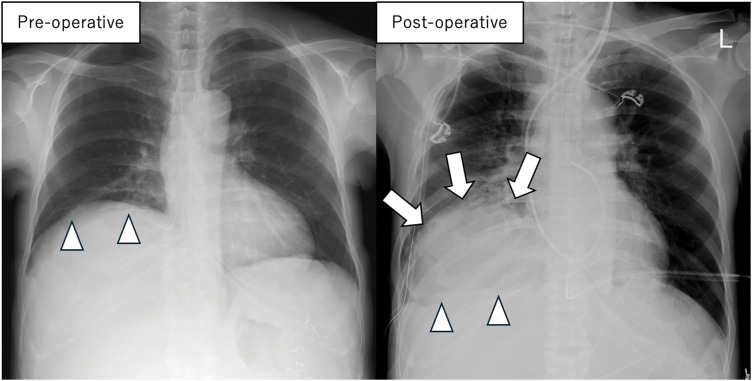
Comparison of preoperative and postoperative chest radiographs. The arrows indicate the pneumatocele and the arrowheads indicate the level of the diaphragm. Postoperatively, a large cystic structure was found to occupy the thoracic cavity.
